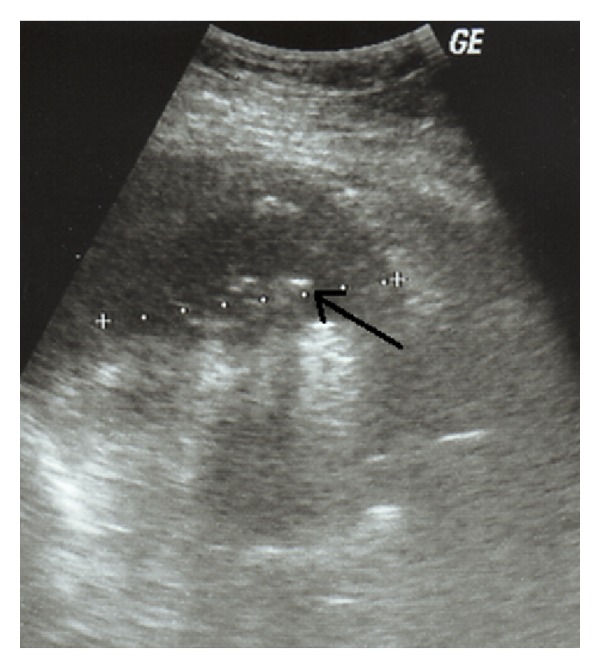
Ultrasound examination showing the presence of a retroperitoneal abscess with hyperechoic foci (spilled gallstones) (arrow).
